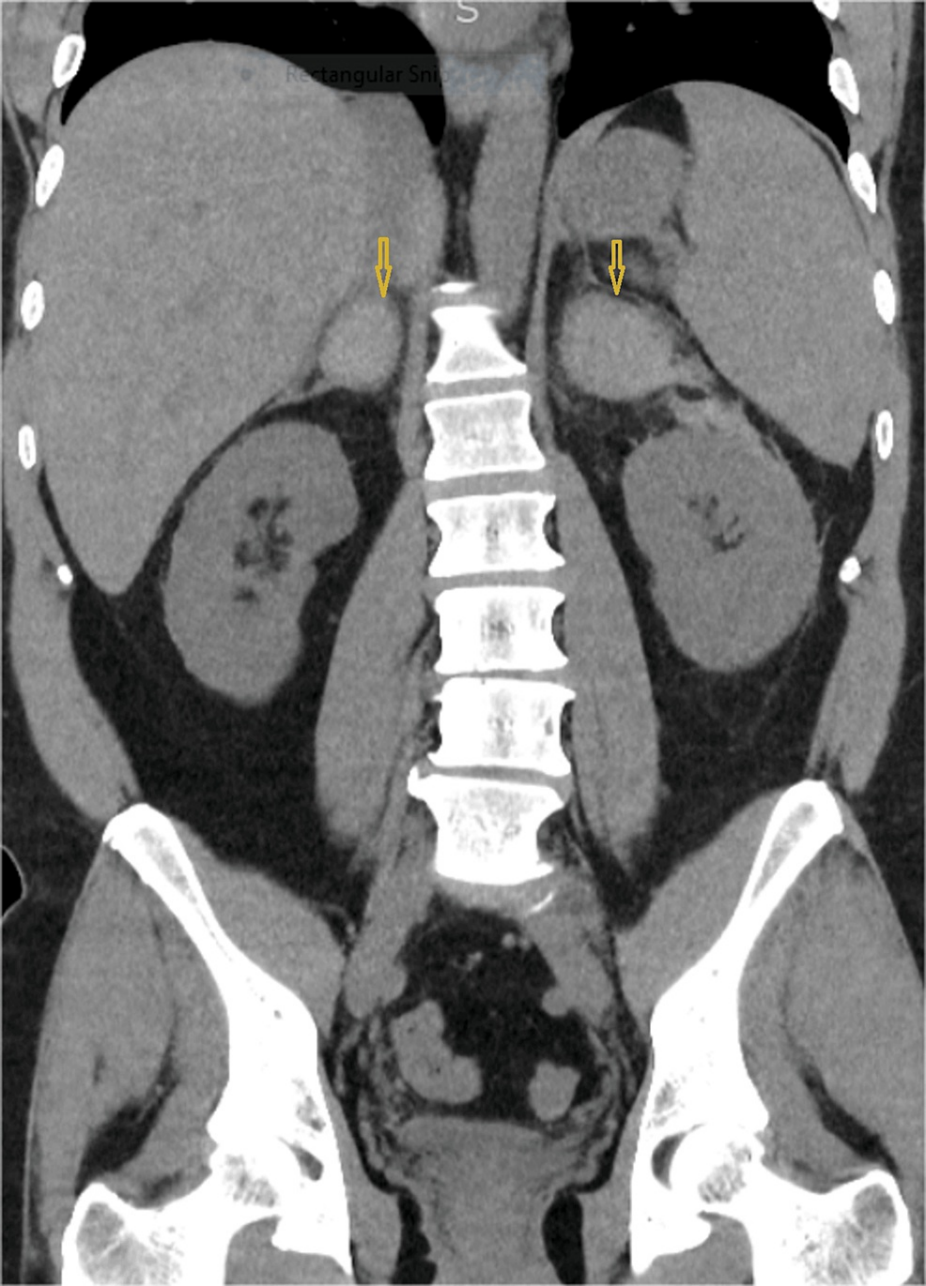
A longitudinal view of the CT scan of the abdomen and pelvis shows bilaterally enlarged adrenal glands (left>right) with adjacent hematoma shown by arrow
